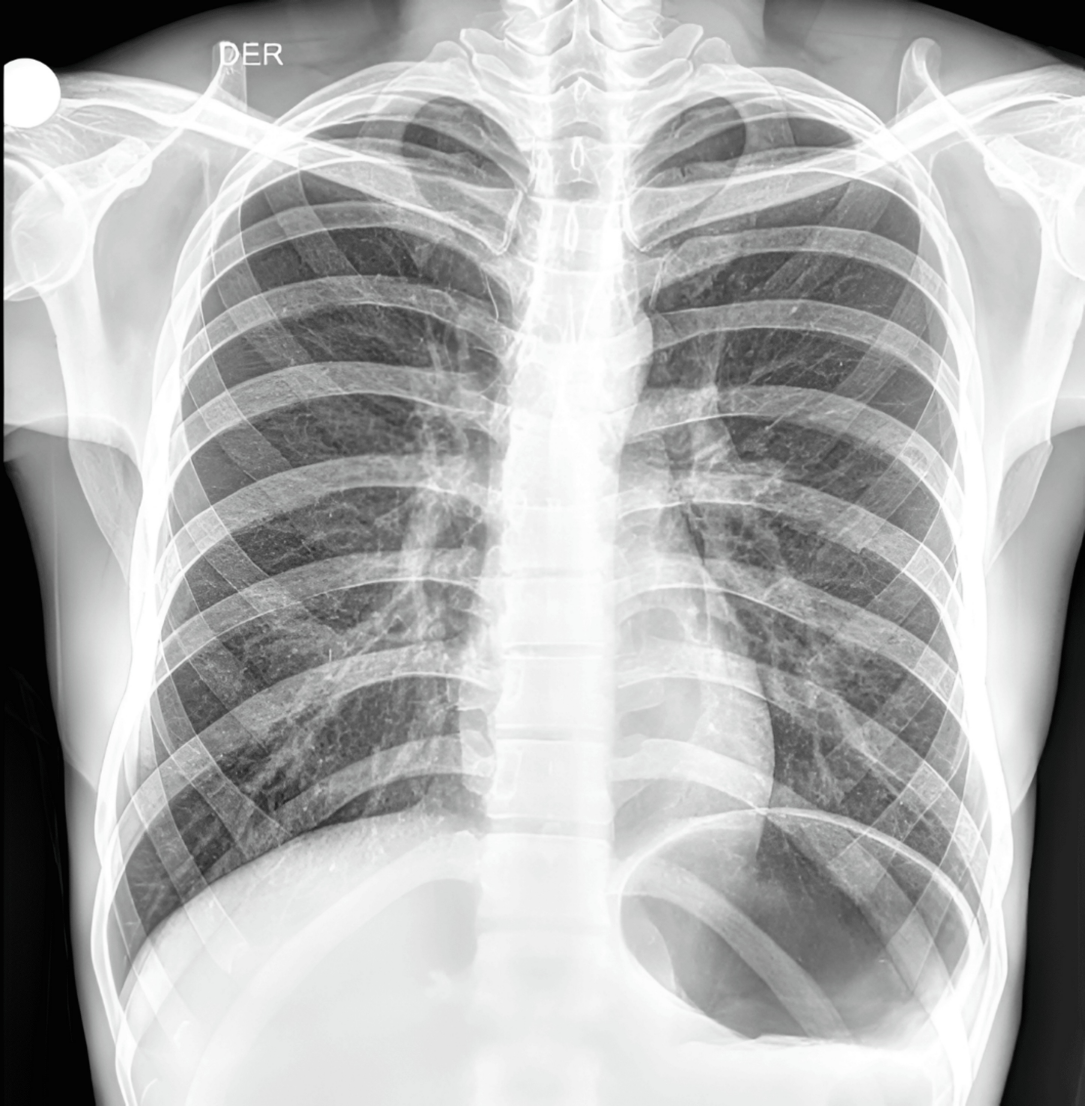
Chest X-ray, posteroanterior (PA) view Posteroanterior chest X-ray demonstrating clear lung fields without infiltrates, cavitations, or mediastinal lymphadenopathy.
